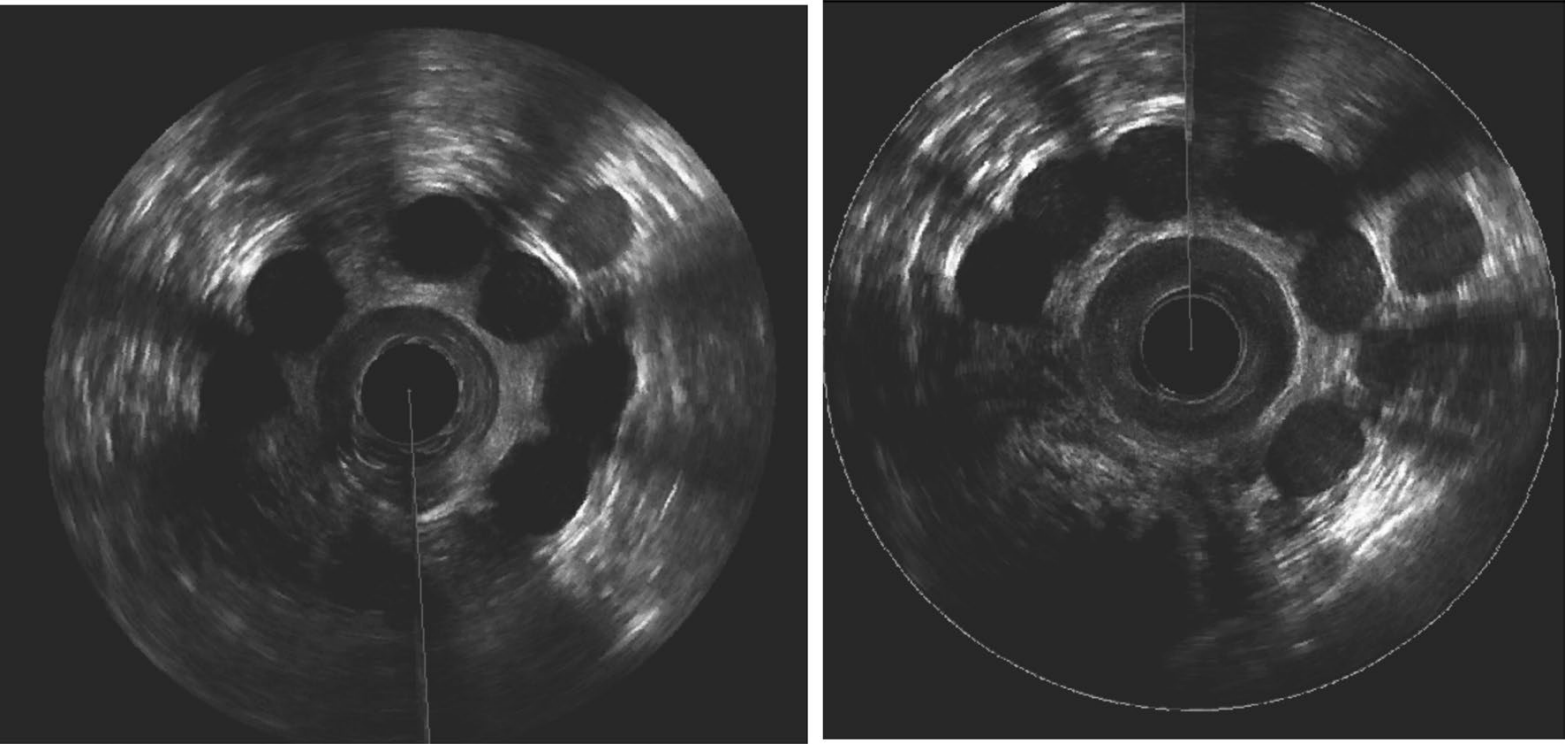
A 3D anal Endosonography 1 month postoperatively: 6 prostheses in the intersphincteric space in correct postion, 1 prostheses migrated outside of the intersphincteric space. B 3D anal Endosonography 16 months postoperatively: 6 prostheses in the intersphincteric space in correct postion, the distace between the prostheses at 10–12’o clock shortened, 1 prostheses migrating inside the intersphincteric space, 1 prostheses sill outside the intersphincteric space
